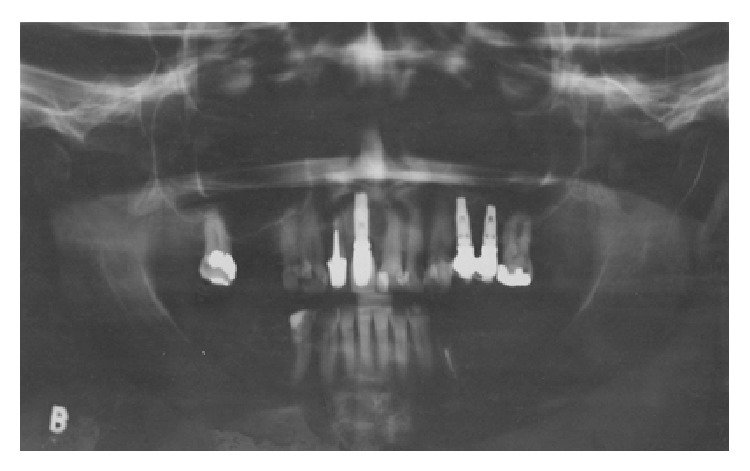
Final panoramic radiography (May 2014).
